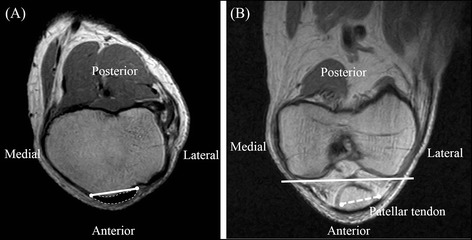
Measurement on MRI. (A) The medial and lateral borders of the patellar tendon at the tibial attachment level (white points) were identified, and the line between these borders was drawn (white line). (B) The most distal points of bilateral femoral condyles were identified, and the line between these points was drawn (white solid line). The line of the patellar tendon was projected (white broken line). The angle between these lines was measured.
